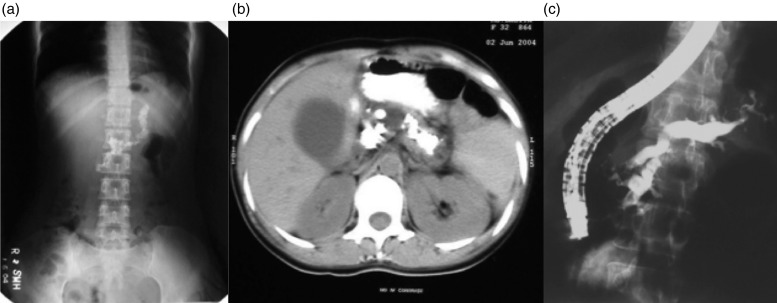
(*a*) Marked pancreatic calcification on a plain x-ray of the abdomen. (*b*) Significant pancreatic atrophy and calculi on a computed tomography scan of the abdomen. (*c*) Dilated pancreatic duct on endoscopic retrograde cholangiopacreatography (ERCP).
